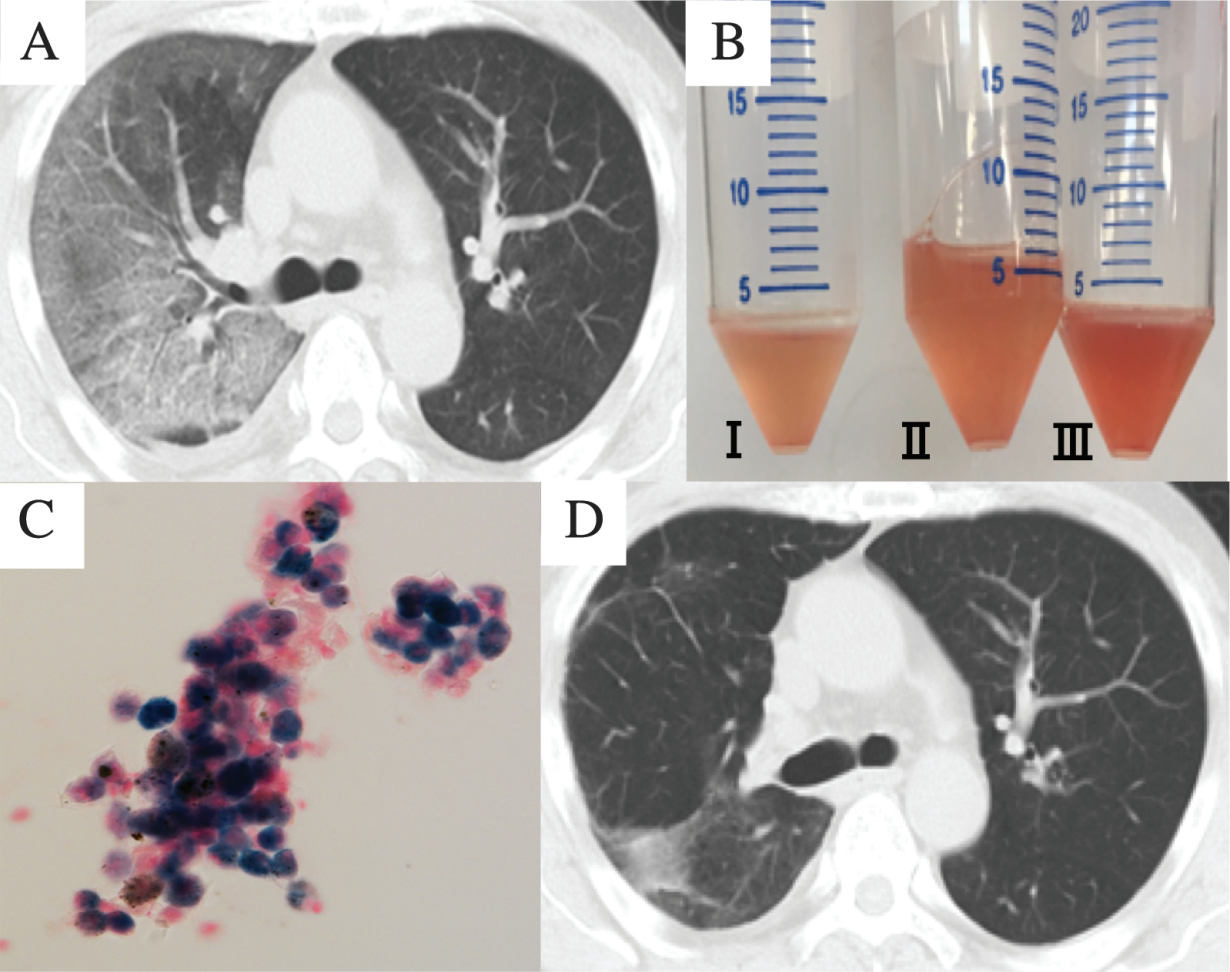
(A) Chest computed tomographic image showing a diffuse ground‐glass opacity in the right upper and middle lobes. (B) The bronchoalveolar lavage fluid (BALF) was haemorrhagic, and a concentration gradient was observed from I to III. (C) Cytological examination of the BALF revealed hemosiderin‐laden macrophages (Prussian blue, 400×). (D) Chest computed tomographic showing complete resolution of the diffuse ground‐glass opacity following discontinuation of methimazole (MMI).
